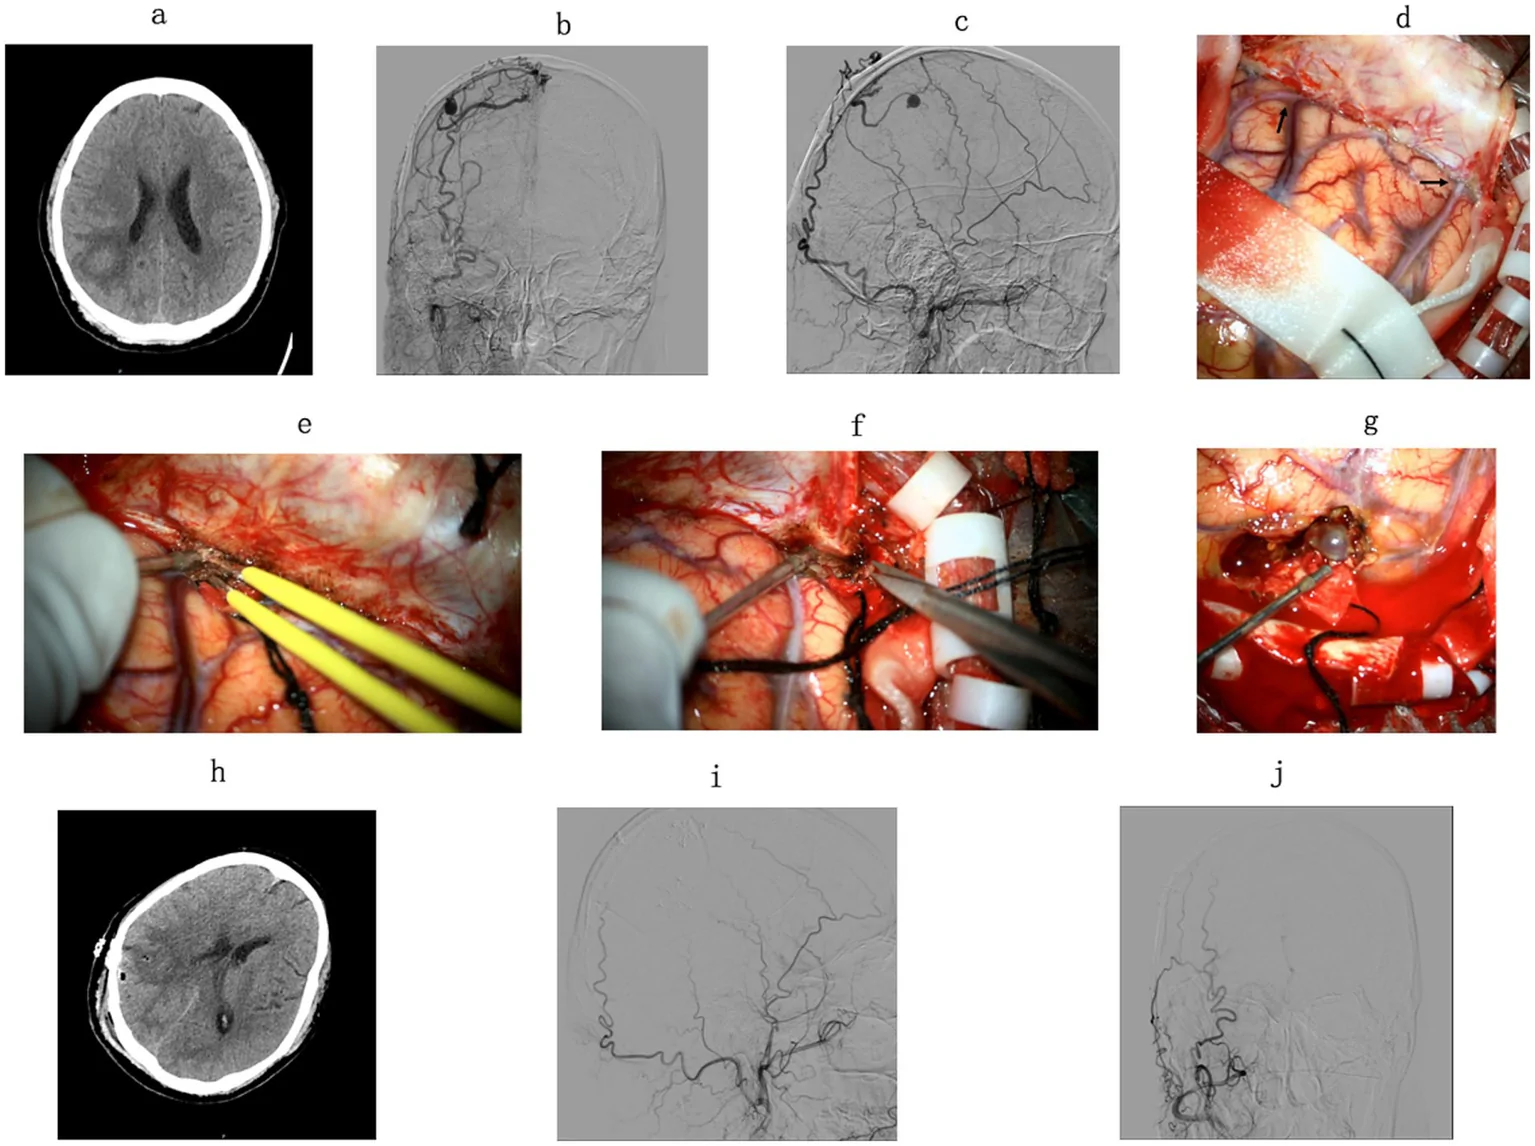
Example of microsurgical treatment for convexity DAVF. (a) Brain computed tomography (CT) reveals an intraparenchymal hemorrhage. (b,c) AP and lateral ECA arteriograms show a convexity DAVF draining into cortical veins. (d) Intraoperative view of the right parietal lobe shows arterialized cortical veins (black arrow). (e–g) Fistula disconnection is completed by coagulating the draining veins distal to the fistulous point. (h) Postoperative non-contrast axial CT confirms coagulation of the venous pouch. (i,j) Six-month follow-up angiograms demonstrate persistent obliteration of the fistula.
